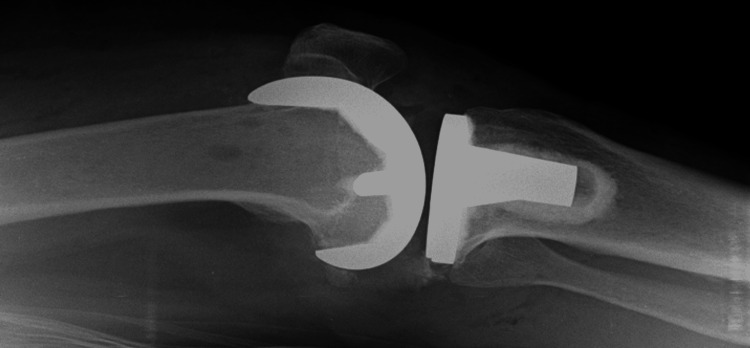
Radiograph with minimum lateral rotation
